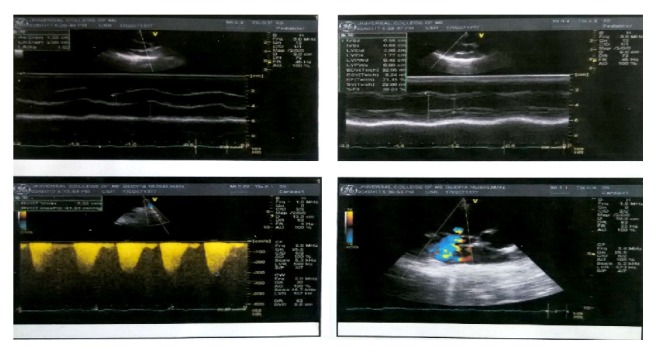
Showing echocardiographic findings.
